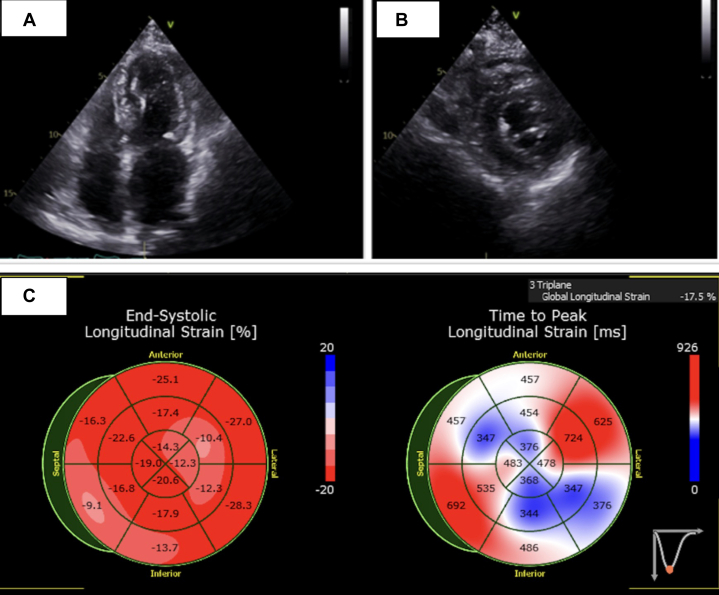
Echocardiogram After Osimertinib Discontinuation Transthoracic echocardiogram demonstrates recovery of left ventricular ejection fraction, evident in the apical 4-chamber (A) and short-axis (B) views. Global longitudinal strain also improved, measuring −17.5% (C).
